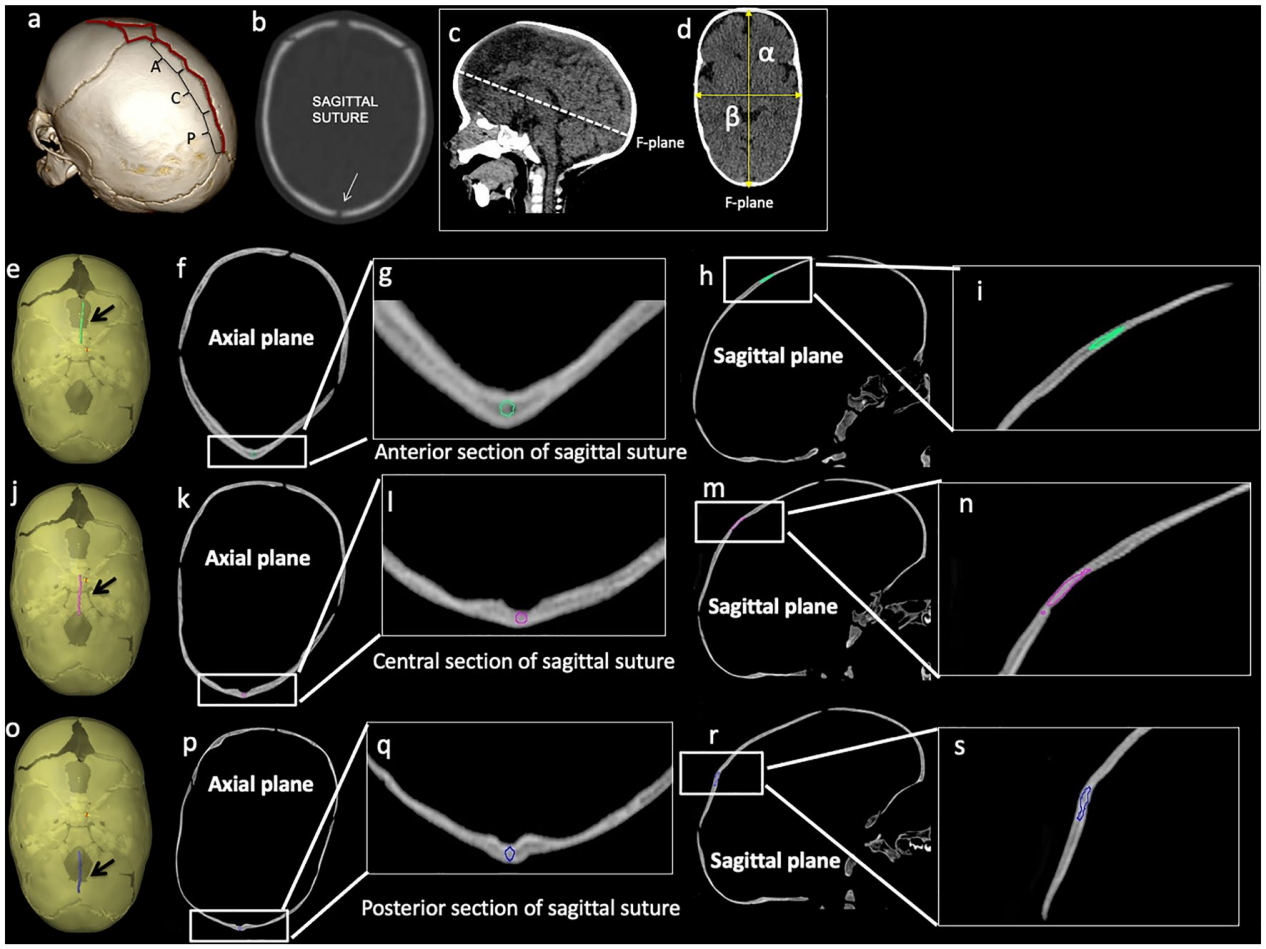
Sagittal suture and skull shape severity quantification using cephalic index. 3D (a) and 2D-CT (b–d). Sagittal suture was subdivided into three sections based on anatomical location (anterior–central–posterior). Patent sagittal suture in b: white arrow. Cephalic index (c, d) represents the ratio of maximum cranial width to maximum cranial length × 100. F plane: the plane at the level of foramina of Monro was used for measurements. Segmentation of sagittal suture on CT images for radiomic features extraction. 3D (e–j–o) and 2D-CT (f, g, h, k, l, m, n, p, q, r, s). Anterior (ROI_anterior: green in e, f, g, h, i; arrow in e), central (ROI_central: pink in j, k, l, m, n; arrow in j) and posterior (ROI_posterior: purple in o, p, q, r, s; arrow in o) section of the sagittal suture. The segmentation of the entire sagittal suture (ROI_entire) is the union of the anterior, central and posterior sections
